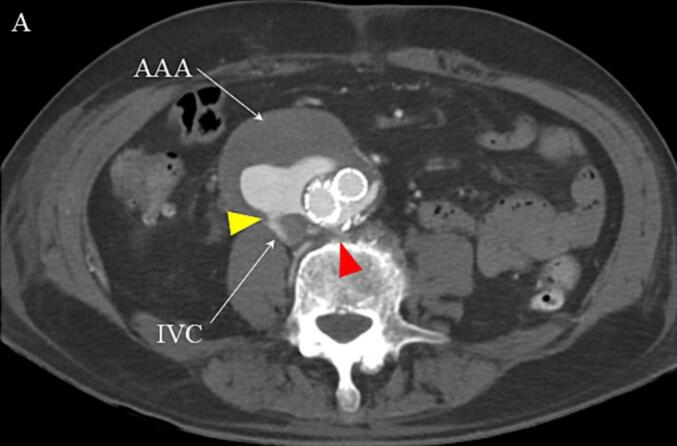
Postoperative Computed Tomography on the Postoperative Day 2. A residual Type II endoleak from the lumbar artery (shown as a red arrow) and reduced aortocaval fistula (shown as a yellow arrow). Abbreviations: AAA, abdominal aortic aneurysm; IVC, inferior vena cava. (For interpretation of the references to colour in this figure legend, the reader is referred to the web version of this article.)
